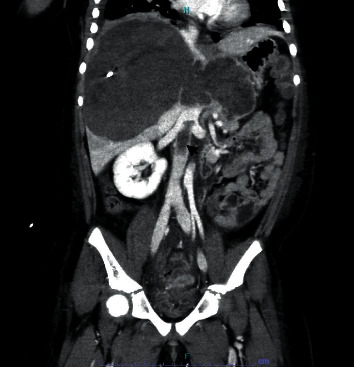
Coronal contrast-enhanced abdominal CT demonstrating IVC thrombus originating adjacent to the right renal hilum.
